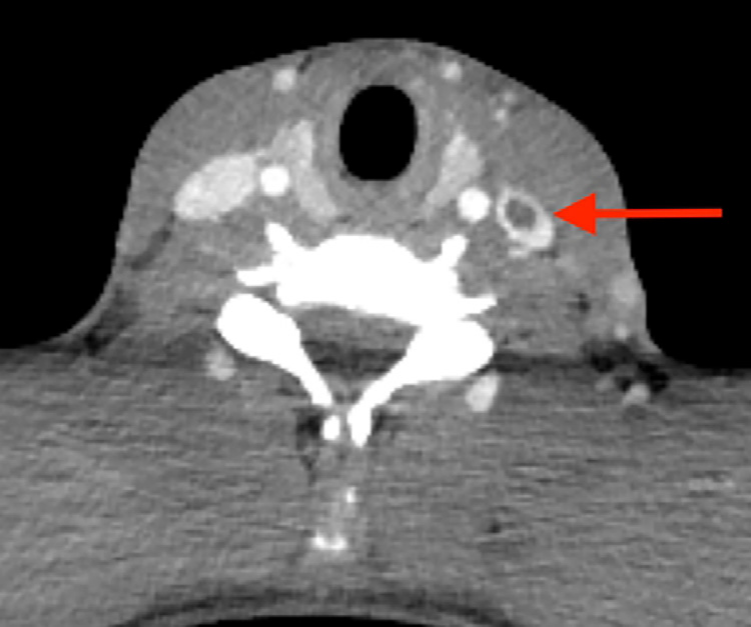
CT pulmonary angiogram mediastinal window: left internal jugular vein with filling defect, representing a thrombus. Of note, a subsequent CT venogram report also identified mild left-sided tonsilitis and further filling defects in the common facial and external palatine veins.
